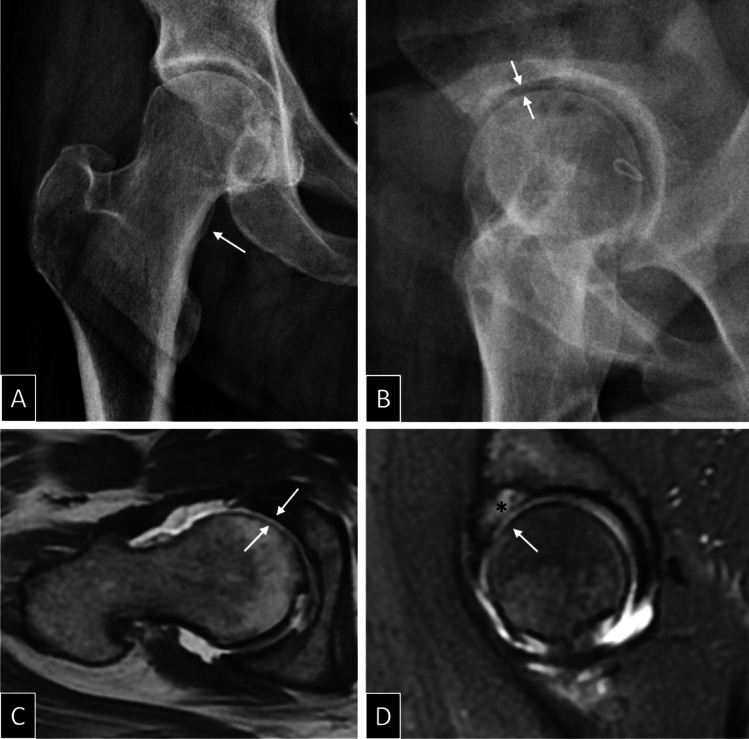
A 38-year-old woman with right hip pain and osteoarthritis. A Anteroposterior radiograph shows cortical buttressing (arrow) with a preserved joint space width (Kellgren 1). B Lequesne false profile shows narrowing of the antero-superior joint space (arrows). C Transverse T1 and D sagittal intermediate-weighted fat suppressed MR arthrography images after intra-articular contrast injection show full-thickness cartilage substance loss in the anterior (arrows in C) and superior (arrows in D) aspect of the joint space, with subchondral cyst-like changes (asterisks in D)
